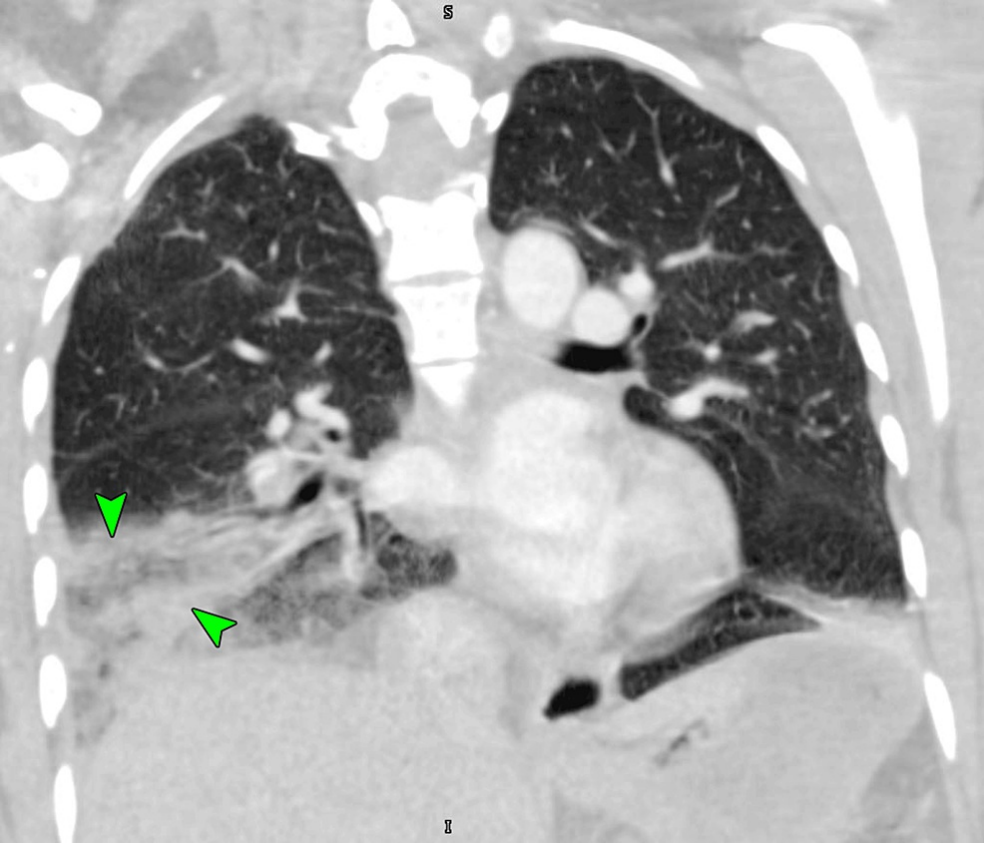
A CT pulmonary angiogram image showing a wedge shaped area of consolidation noted in the right lower lobe, representing a pulmonary infarct (arrowheads) CT: computed tomography
